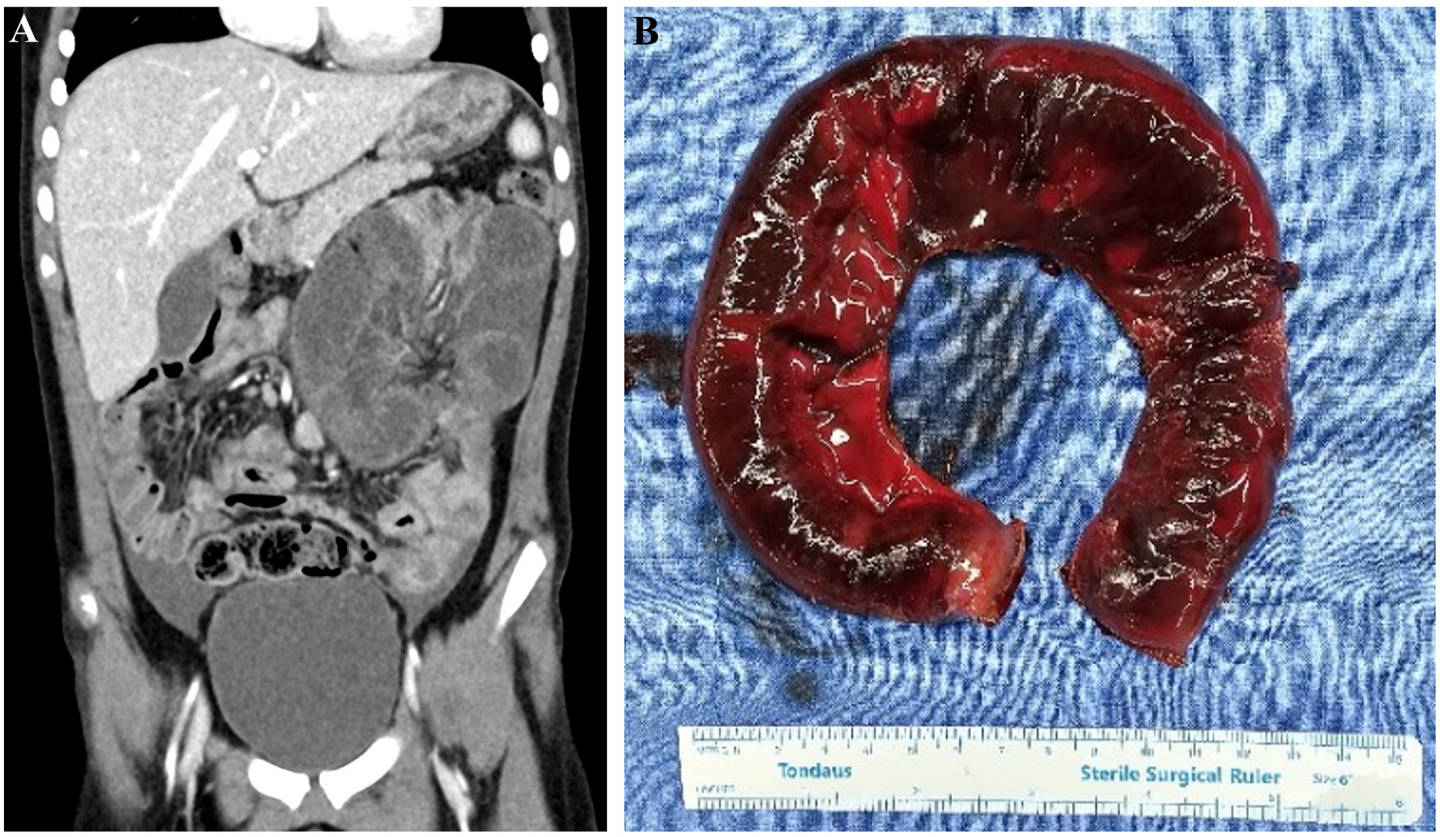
Imagings before and during 3rd surgery. (A) CT scan showing marked distension of small bowel loops and decreased enhancement, indicating closed loop obstruction with ischemia. (B) Intraoperative image of resected jejunum. Apart from ischemia, the diffuse circular stenoses found in the previous operations seemed almost disappeared.
